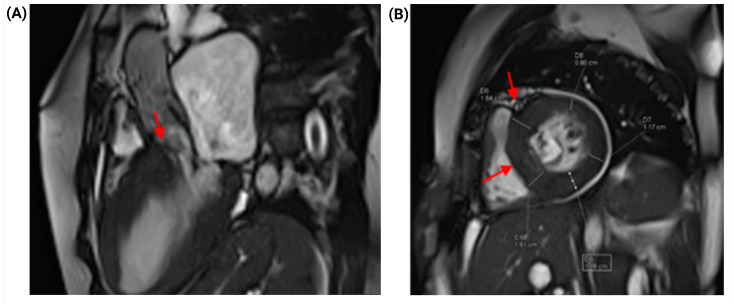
Cardiac magnetic resonance imaging: structural characterization and myocardial fibrosis. (A) Cardiac magnetic resonance image demonstrating an elongated subaortic membrane (red arrow) consistent with fixed subvalvular LVOT obstruction at rest. (B) Short-axis cardiac magnetic resonance image showing asymmetric hypertrophic cardiomyopathy with predominant septal hypertrophy (maximum septal thickness 16.4 mm; red arrows) and myocardial tissue characterization demon-strating late gadolinium enhancement, quantified as 13% myocardial fibrosis.
